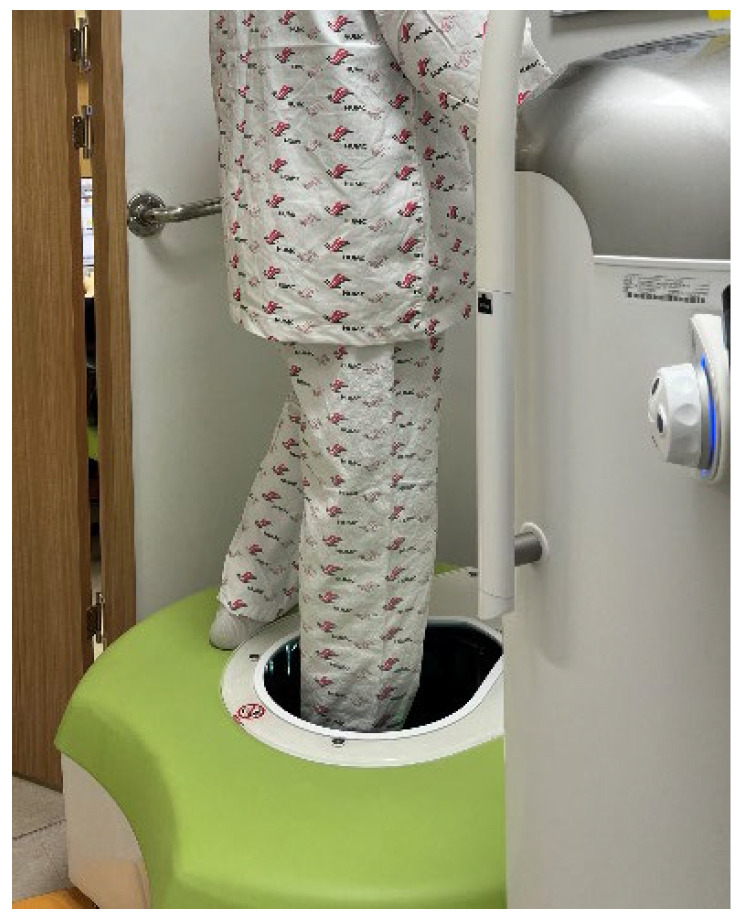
This photograph showed the position during upright weightbearing foot examination using a cone-beam CT scanner. Scanner design with flexible gantry movements allows weightbearing imaging in a standing position.
